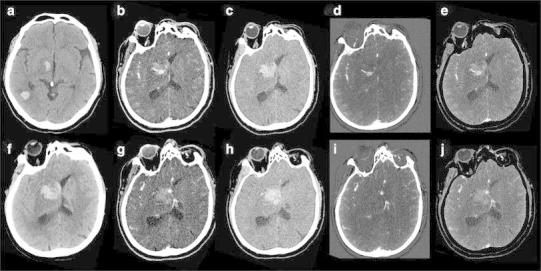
65-year-old male presented with left-sided hemiparesis due to ICH. The patient deteriorated within 1 h and a DECTA/DECTV was performed. The patient was non-cooperative at DECTA as demonstrated by the rotated head. NECT (*a*—at presentation, *f*—1 h later), mixed images (H31 kernel) (*b*, *g*), VNC (*c*, *h*), IOM (*d*, *i*), and bone removal (*e*, *j*) from DECTV are shown. The hematoma is expanded in between the two conventional CT scans. Two extravascular enhancing lesions are present at the mixed, IOM, and bone removal images, consistent with spot signs. The VNC clearly demonstrates the hematoma at lower SNR but still rated as diagnostic. All reconstructions, even bone removal, from DECTA can be made, despite the relative non-cooperation of the patient (conventional CT (*a*) 120 kVp, 310 mAs, CTDI 69.44 mGy, DLP 1062 mGycm. Conventional CT (*f*) 120 kVp, 285 mAs, CTDI 48.28 mGy, DLP 833 mGycm. DECTV 80/Sn140 kVp, 310/155 mAs, CTDI 28.86 mGy, DLP 520 mGycm; 95 cc iodinated contrast 300 mg/ml; injection rate 5.5 cc/s; 40 cc saline flush 5.5 cc/s, delay 45 s)
